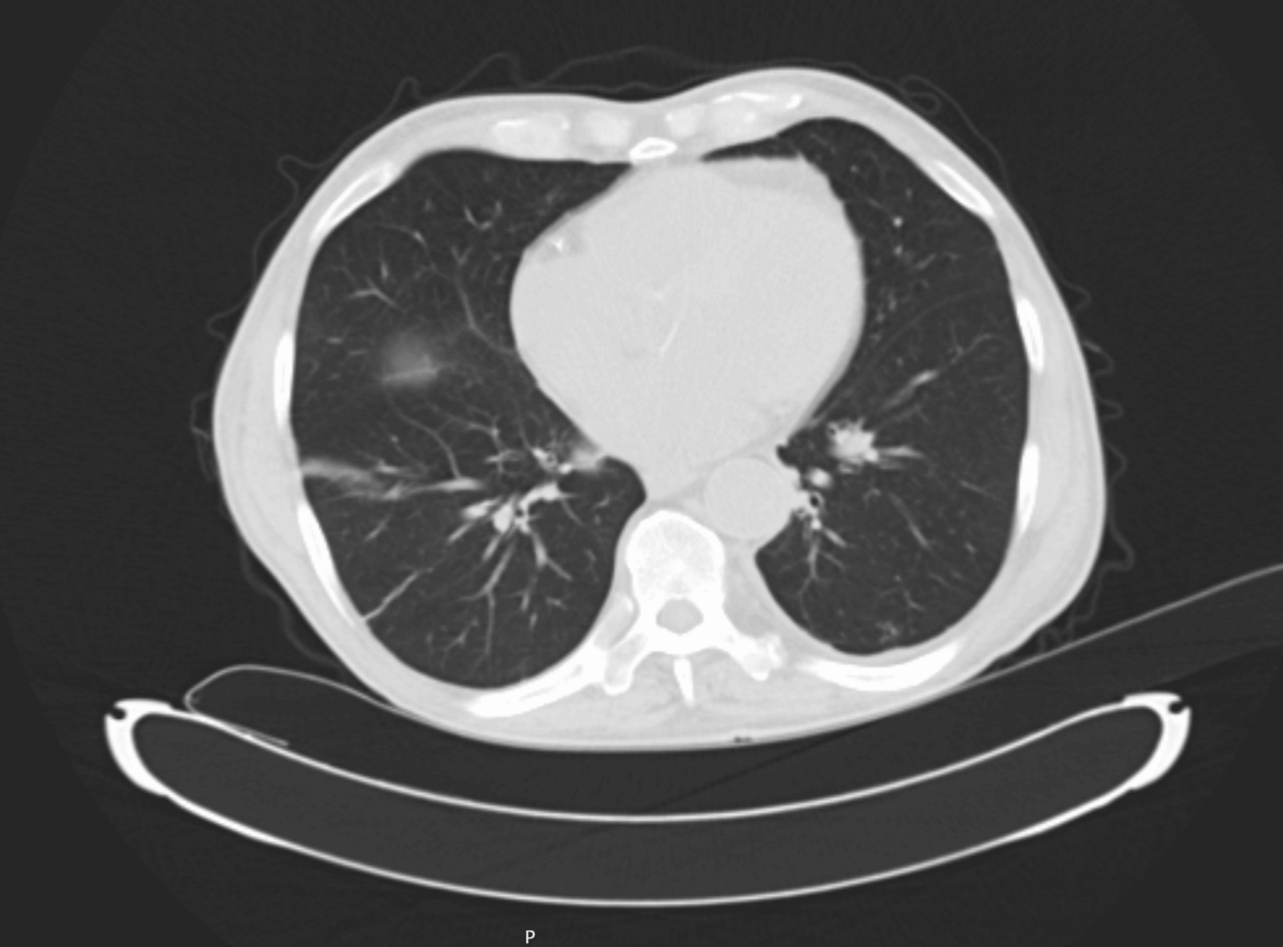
Chest computed tomography findings Axial chest computed tomography demonstrating pleuropulmonary fibrotic changes and pericardial effusion.
